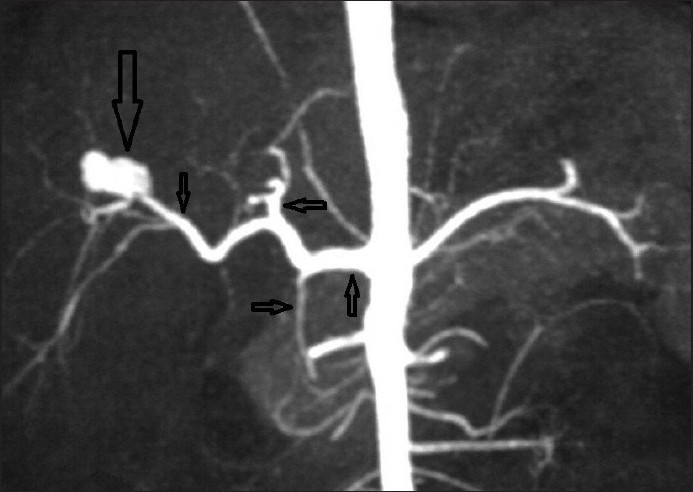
Coronal plane thick multiplanar reformation CT angiography image showing right hepatic artery pseudoaneurysm with normal common hepatic artery (upward arrow), left hepatic artery (leftward arrow), and gastroduodenal artery (rightward arrow); proximal right hepatic artery is normal (small downward arrow) with pseudoaneurysm arising further distally (large downward arrow)
